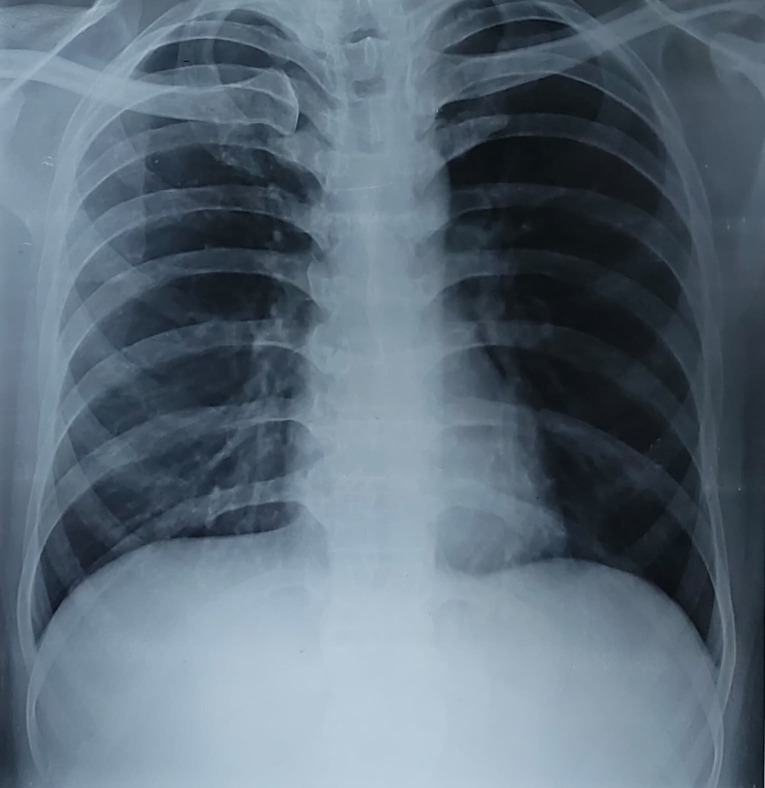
Chest X-ray (posterior–anterior view) showing no evidence of active or old tuberculosis.
